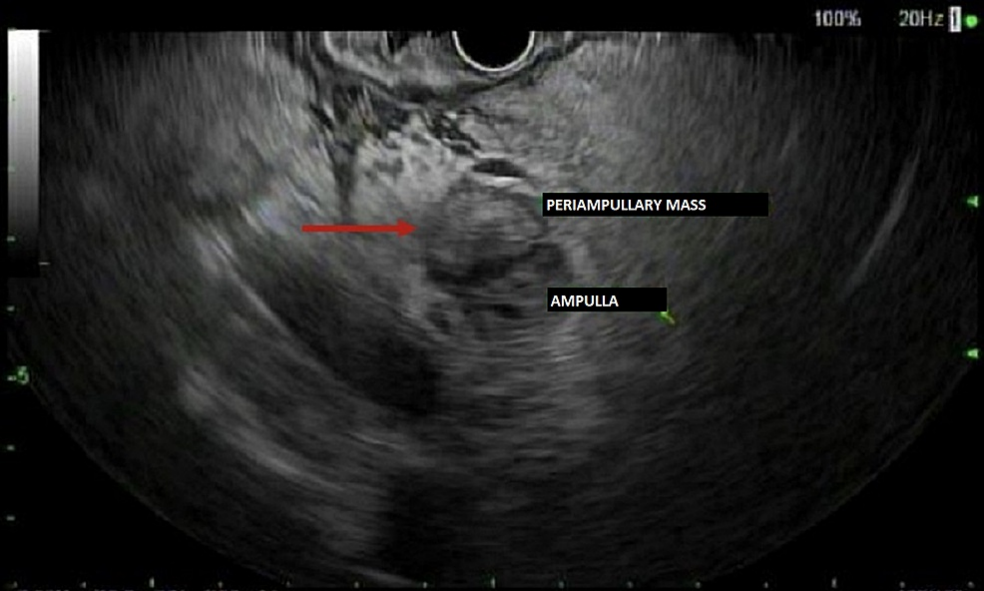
EUS showing periampullary diverticulum EUS - endoscopic ultrasound
